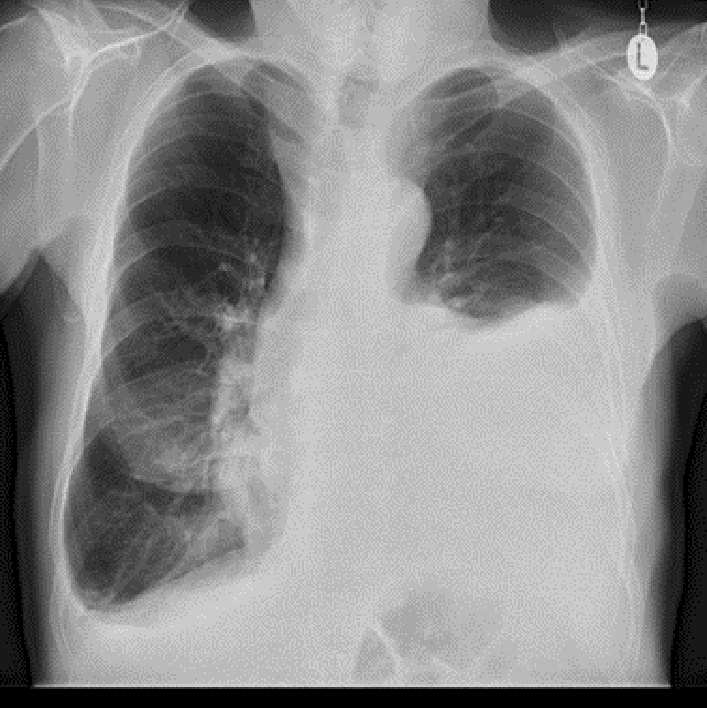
Chest X-ray—posteroanterior view
